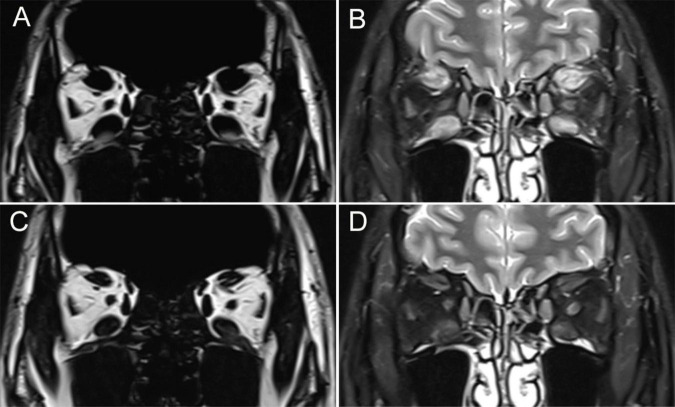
Representative MRI findings from one TED patient revealed an improvement in EOM enlargement and edema following radiotherapy. This patient is a 55-year-old male with a 9-month history of TED. Before radiotherapy, coronal MRI demonstrated enlargement of the EOM on both T1- and T2-weighted imaging, along with increased T2 signal intensity (A,B). Six months after radiotherapy, this patient showed a decrease in EOM enlargement and T2 signal intensity (C,D).
